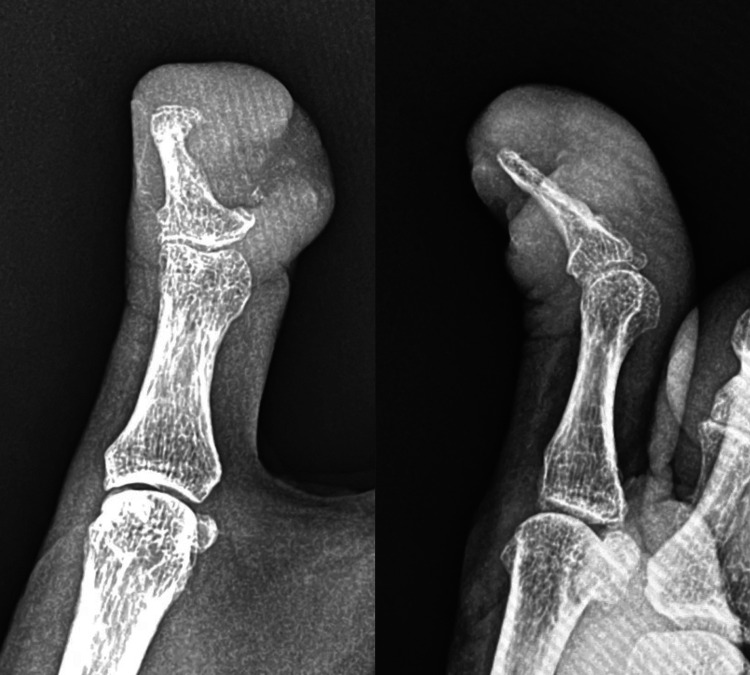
Preoperative radiographs (left: frontal view, right: lateral view).
